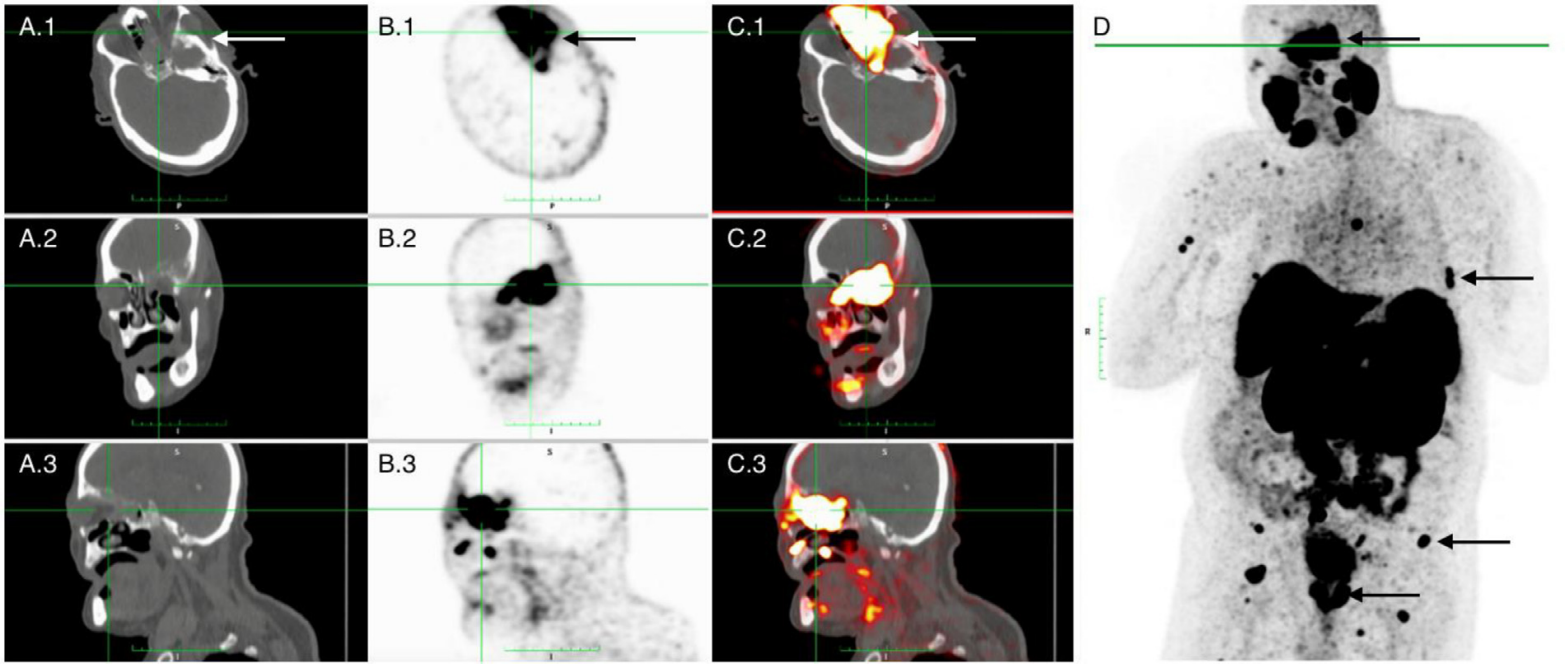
Axial, coronal and sagittal CT (A.1-A.3) and axial, coronal and sagittal PET images (B.1-B.3) revealed an intense PSMA focus in the left orbit with soft tissue involvement on CT (arrow). Fused PET/CT imaging by ^18^F-PSMA-1007 displayed the orbital lesion with intense tracer activity (arrow; C.1-C.3). Additionally, the maximum intensity projection (MIP) of ^18^F-PSMA-1007 PET/CT (D) showed the PSMA-avid orbital lesion (arrow 1), extensive bone metastases throughout the body (arrow 2), strong PSMA uptake in multiple (loco)regional lymph nodes (arrow 3), and intense tracer expression in both sides of the prostate with invasion of the left seminal vesicle (arrow 4).
